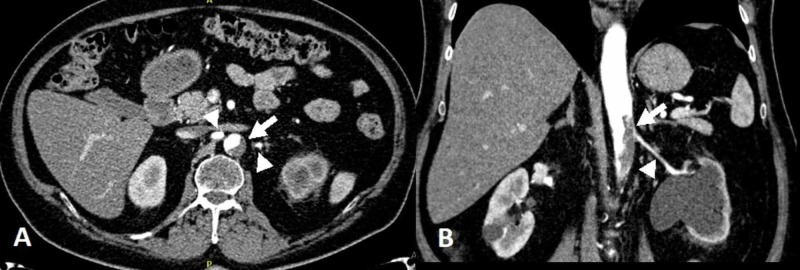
Enhanced computed tomography scan during the arterial phase (A) Axial view at the level of the takeoff of the renal arteries, revealing an aortic mural thrombus (arrow) almost obliterating the ostium of the left renal artery. Both renal arteries seemed opacified (arrowheads); (B) Coronal view showing again the mural aortic thrombus (arrow) at the level of the renal artery and extending caudad. Also noted here is the left renal artery (arrowhead), left hydronephrosis, and decreased uptake of the contrast due to effaced kidney parenchyma, unlike the contralateral right kidney.
